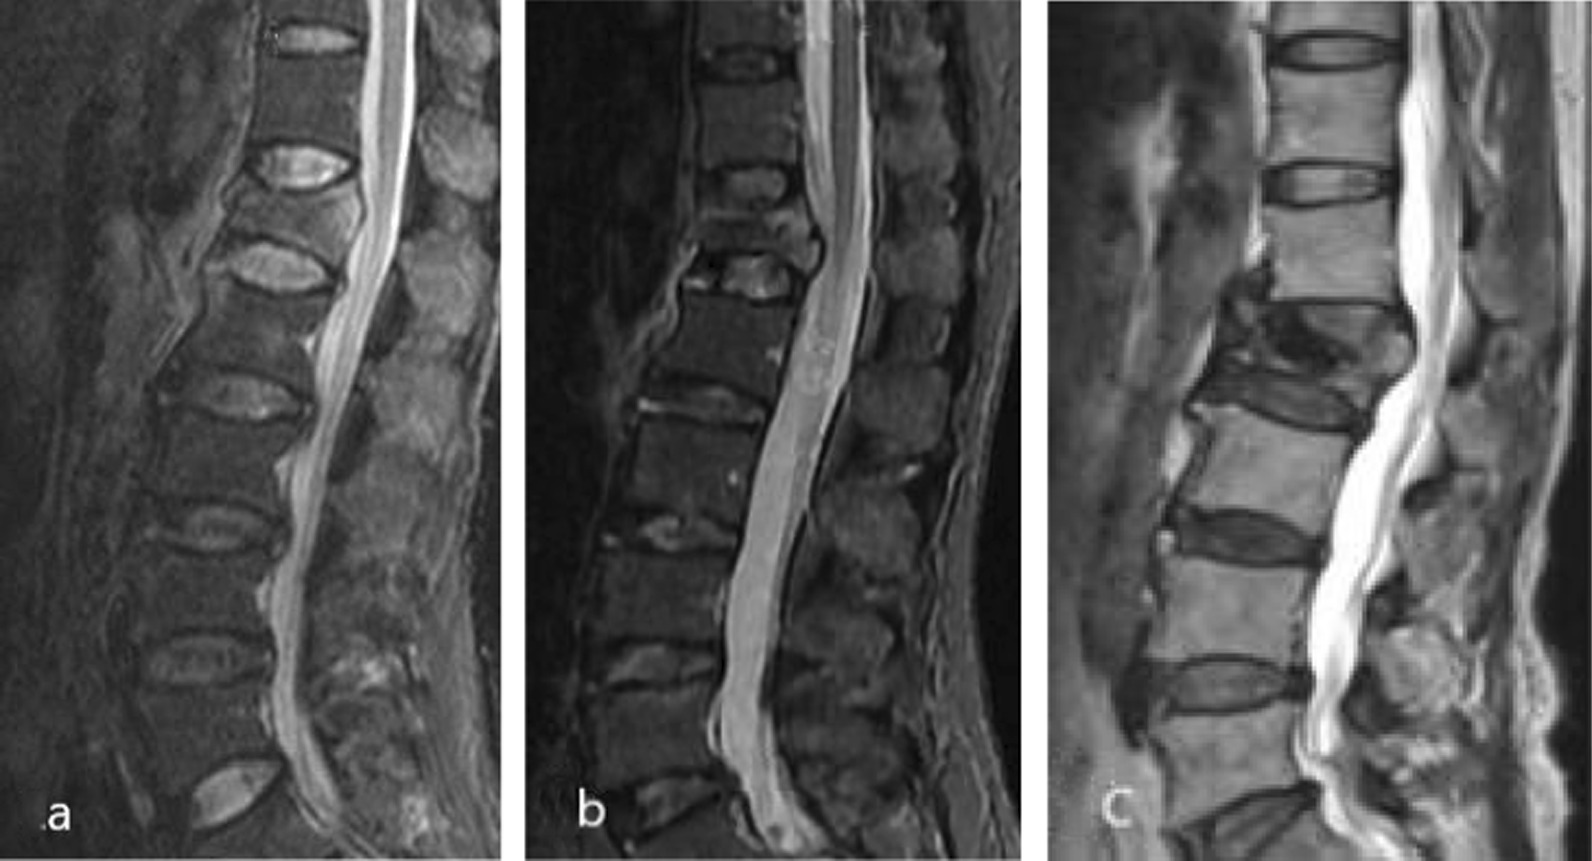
Pre-treatment thoracolumbar MRI. According to the imaging features of intervertebral disc injury, it was divided into three categories: no injury, mild injury, and moderate-to-severe injury. a Intervertebral disc was not damaged (0 point); b mild injury of intervertebral disc, signal change, no endplate injury, and with or without gap change (1 point); and c intervertebral disc moderate-to-severe injury, signal changes, endplate rupture, intervertebral disc content hernia into the vertebral body, and intervertebral space change (2 points)
